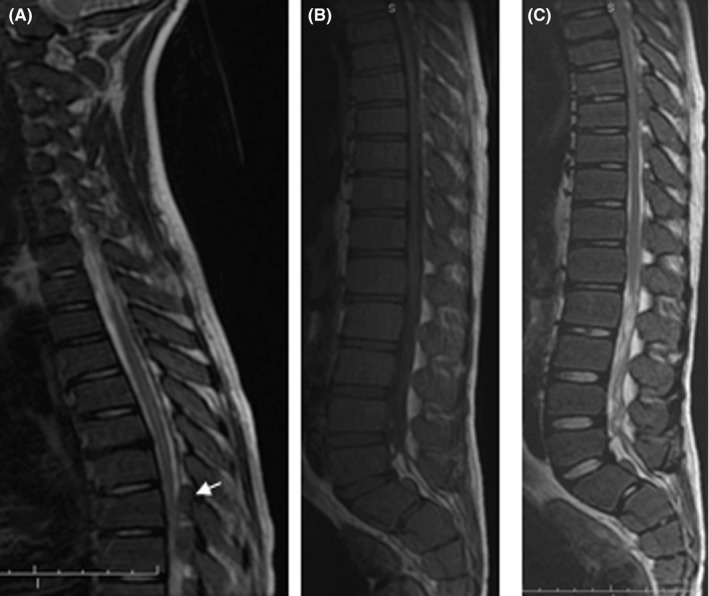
MRI sagittal T2 and T1 WI demonstrated significant improvement of the epidural masse
